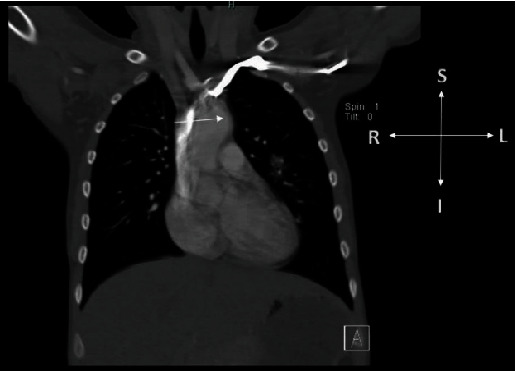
Initial chest CT, coronal view demonstrates what was believed to be a dissection flap (arrow) extending superiorly from the level of the anterior cusp of the aorta.
